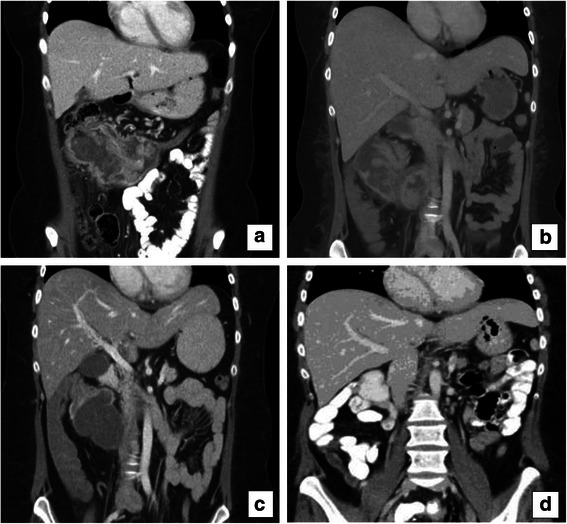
A computed tomography (CT) with contrast before treatment (a), 2 months after FLOX treatment (b), 4 months after treatment with metronomic capecitabine/bevacizumab (c) and 9 months after surgery (d)
